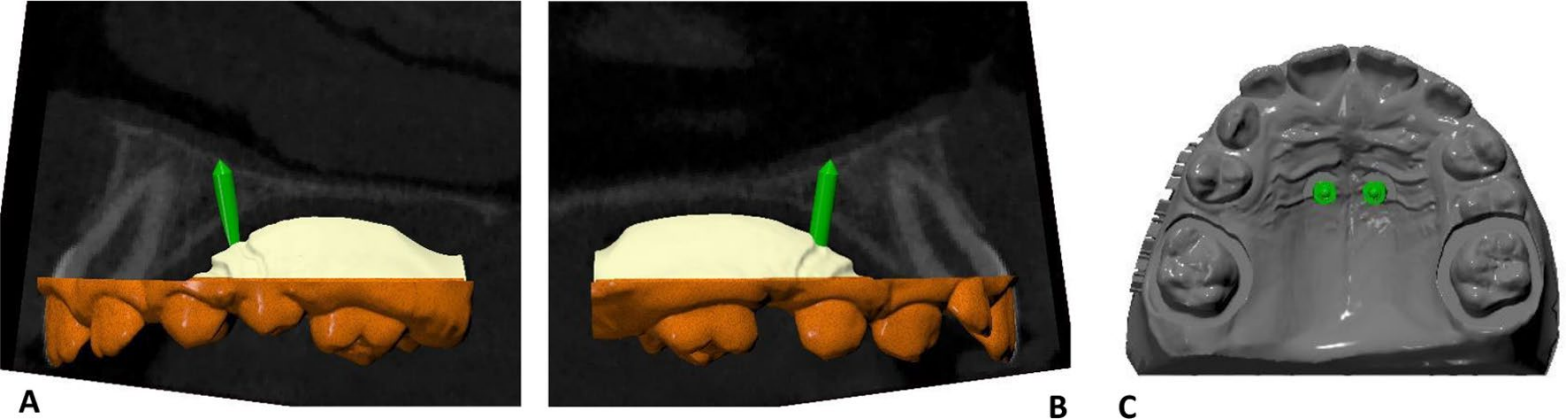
Digital planning of bicortical insertion of two paramedian miniscrews using the REPLICA System®. A, B virtual miniscrews position on the superimposition of cone-beam computed tomography and digital impression. C coronal view of the position of the virtual miniscrews on the digital model
